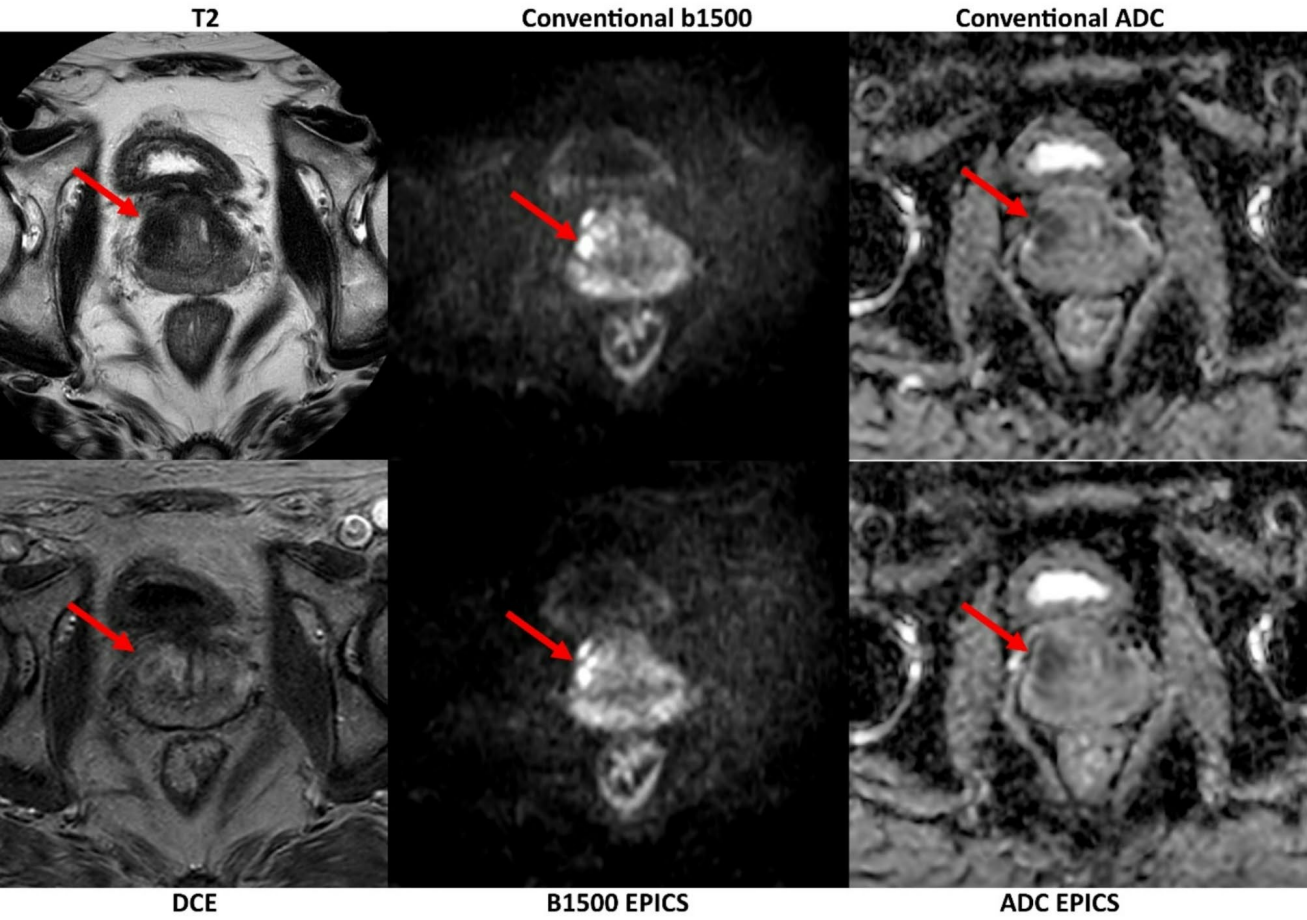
T2 image, dynamic contrast-enhanced (DCE) image, as well as DWI b1500 images and apparent diffusion coefficient (ADC) maps obtained from conventional diffusion-weighted images (DWI) and accelerated DWI sequences using echo planar imaging and compressed SENSE based reconstruction (EPICS). A PIRADS 5 lesion is shown in the right mid anterior transitional zone (red arrows).
